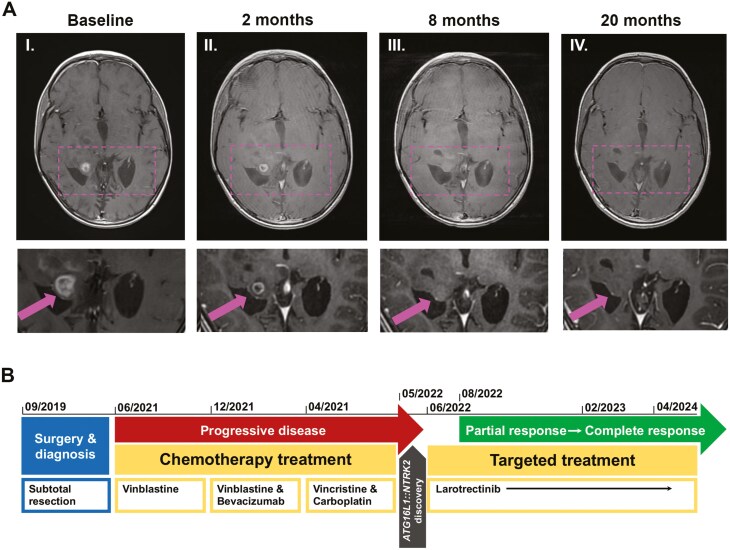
Treatment results update. (A) MRI as per RANO from baseline to last follow-up in the SCOUT clinical trial (upper panel: whole head MRI view; lower panel: zoomed in MRI view). (I) Baseline, June 2022, before the start of larotrectinib treatment, presenting with one target measurable contrast-enhancing lesion on the right thalamus (shown in the image) and 3 nonmeasurable contrast-enhancing lesions (not shown). (II) The first follow-up MRI image, August 2022, after 2 cycles of larotrectinib, showing a decrease in size of the measurable enhancing lesion ≥ 50% from the baseline MRI scan. No progression of the nonmeasurable lesions (not shown) and no new lesion occurrence was observed. The RANO imaging tumor status was a partial response. (III) In February 2023, after 8 cycles of larotrectenib, the target measurable contrast-enhancing lesion, as well as the 3 nonmeasurable contrast-enhancing lesions, were no longer detectable, and no new lesion occurrence was observed, consistent with a radiological tumor status of complete response. (IV) At the last MRI follow-up in April 2024, after 1 year and 10 months, the RANO imaging tumor status of complete response was sustained. (B) The timeline for tumor development/status and the patient’s treatment history from diagnosis in September 2019 up to the last follow-up in April 2024.
